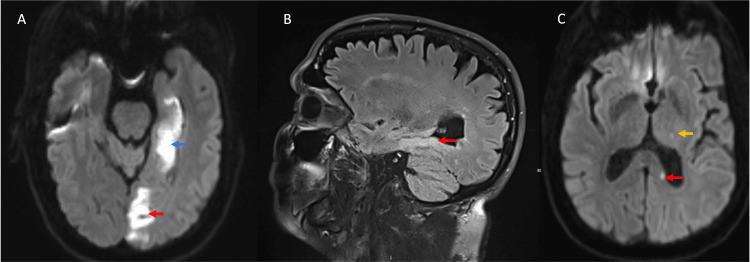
Case 2: Acute ischemic stroke in the left PCA territory MRI shows involvement of the temporal lobe (panel A, blue arrow), occipital lobe (panel A, red arrow), parahippocampal gyrus (panel B, red arrow), the thalamus (panel C, yellow arrow), and splenium of the corpus callosum (panel C, red arrow). PCA, posterior cerebral artery
